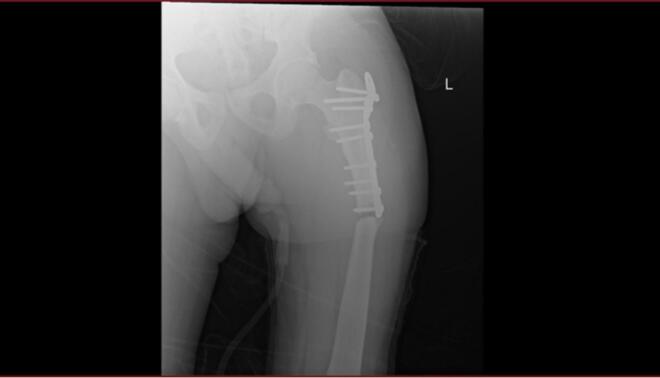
AP radiograph of the left femur showing the previous plate and screws with subtrochanteric fracture.
